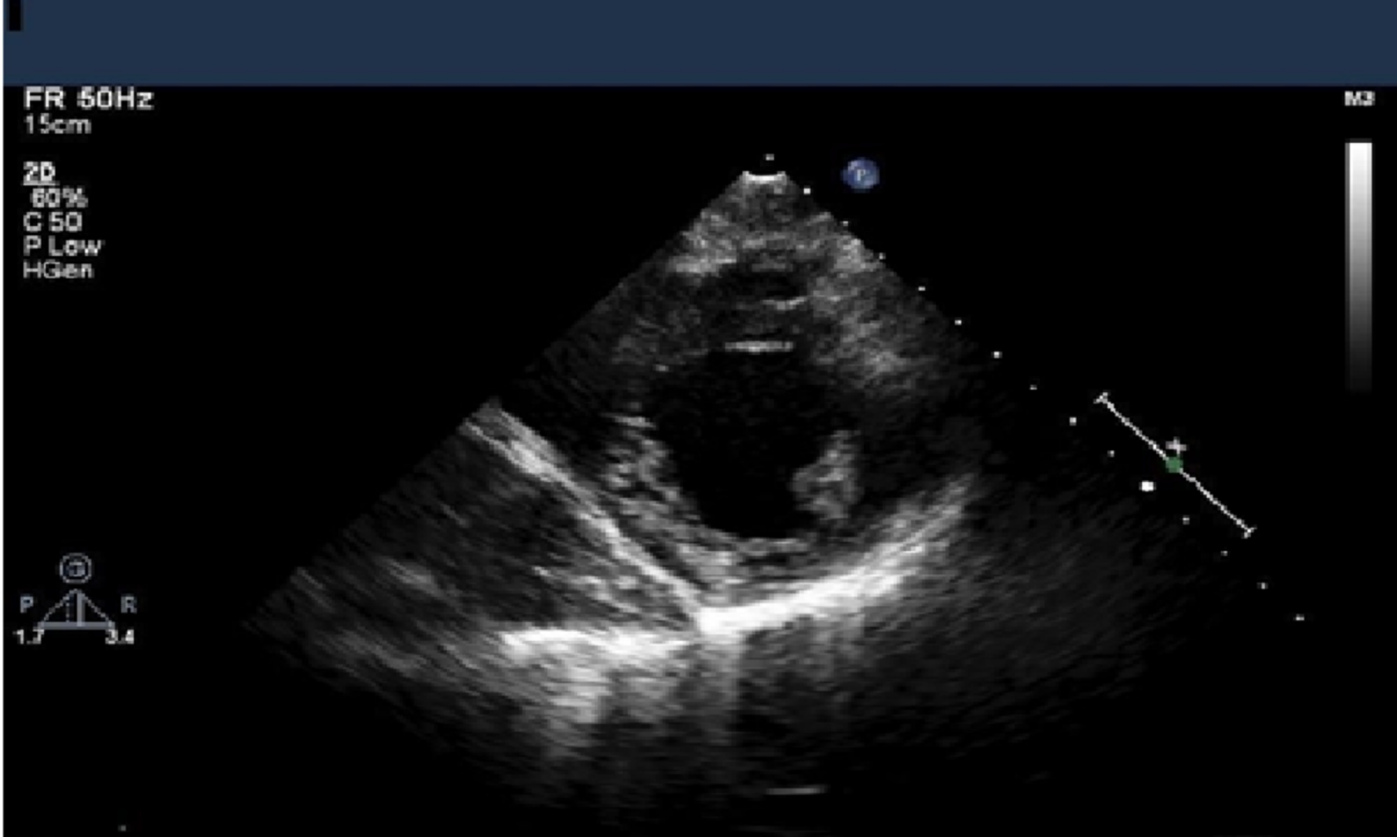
Short axis parasternal view showing a resolved pleural effusion
